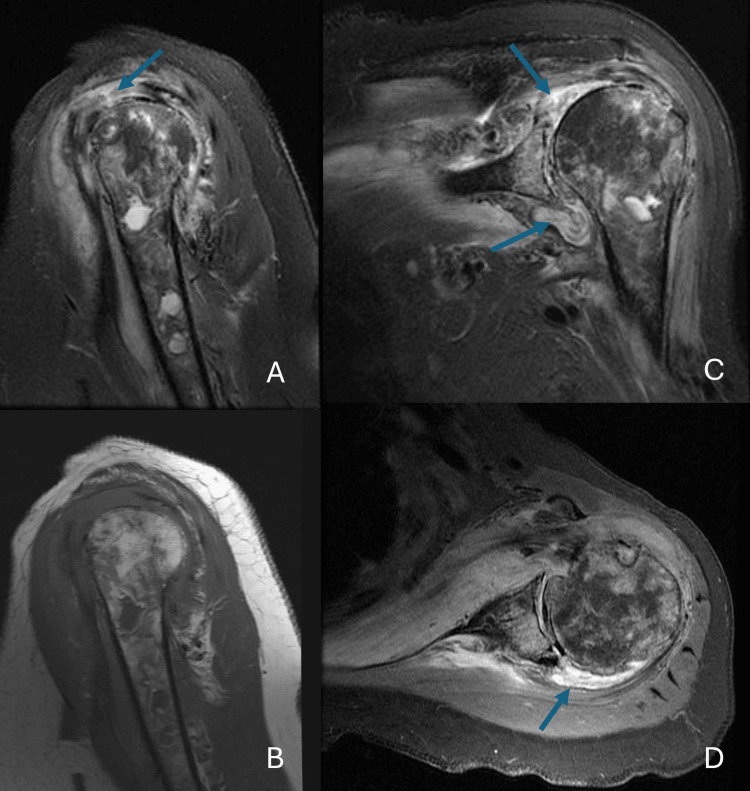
MRI of left shoulder sagittal T2 FS (A) with arrow at subacromial-subdeltoid bursitis, sagittal T1 (B), coronal PD FS (C), and axial PD FS (D) demonstrating heterogeneous appearance of humerus consistent with osteomyelitis and arrows demonstrating joint effusion and bursitis FS, fat saturation; PD, proton density
